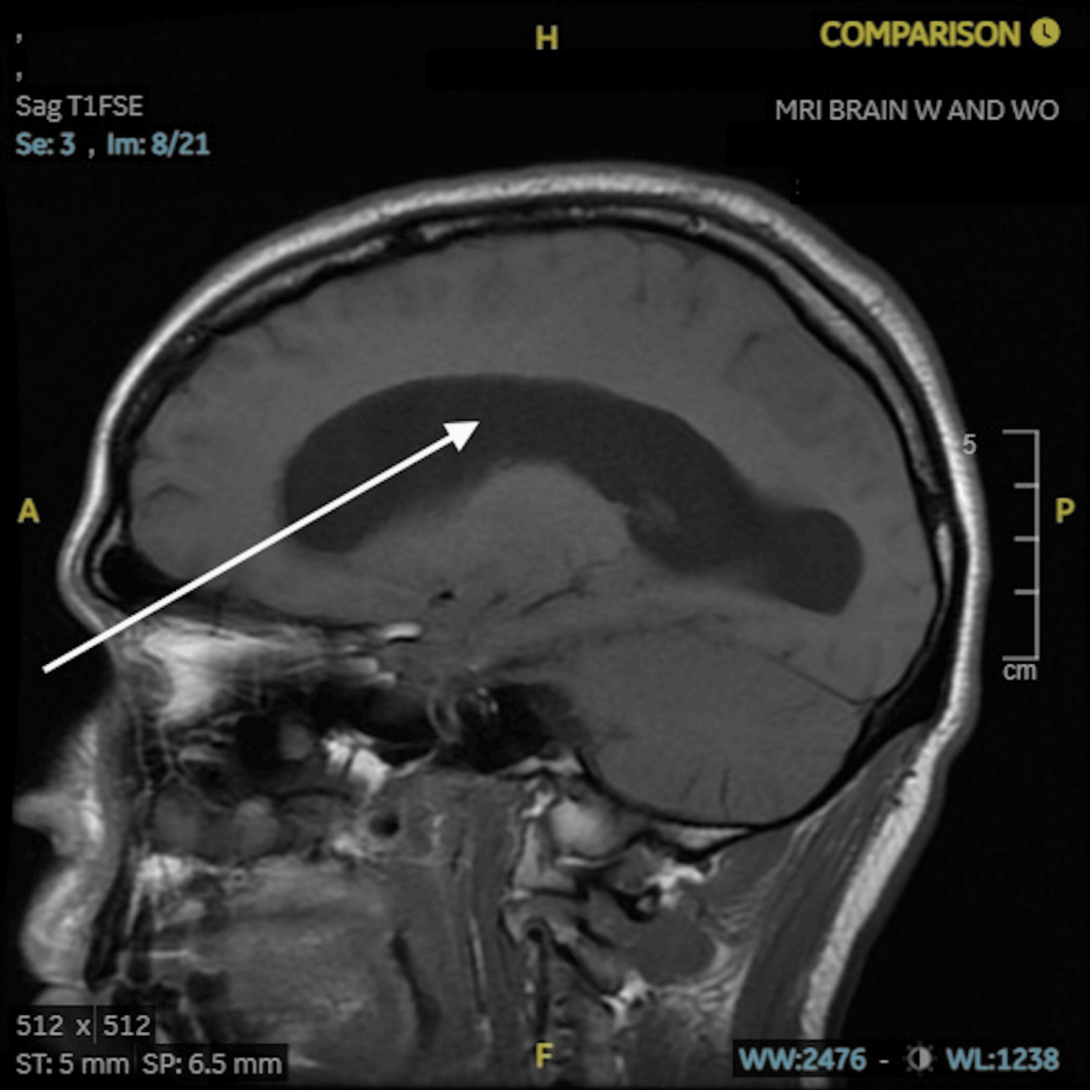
MRI without contrast sagittal view T1-weighted, demonstrating dilated ventricles and hydrocephalus MRI: magnetic resonance imaging
